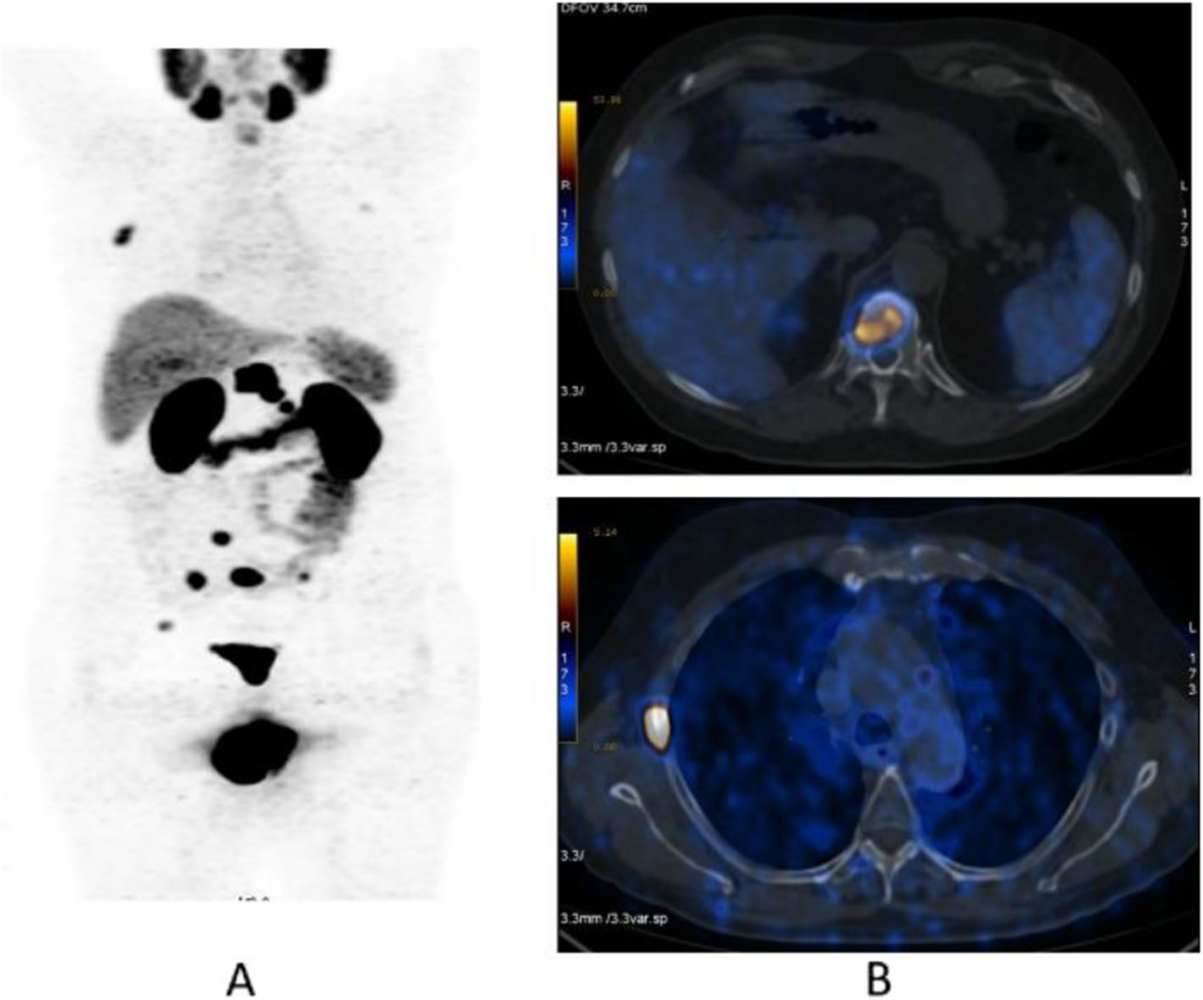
^68^Ga-PSMA, prostate cancer, biochemical recurrence (BCR): 223Ra feasability. *Clinical history*: 72 y.o. man with prostate cancer, radical prostatectomy as primary treatment Gleason score 4 + 3; during abiraterone treatment, PSA increased up to 127 ng/ml, candidate to 223Ra chloride. *PET/CT findings*: multiple foci of increased uptake in the bones. No lymph node or visceral metastases. After ^68^Ga-PSMA PET/CT, the patient has been referred to 223Ra chloride treatment. a MIP; b fused images
